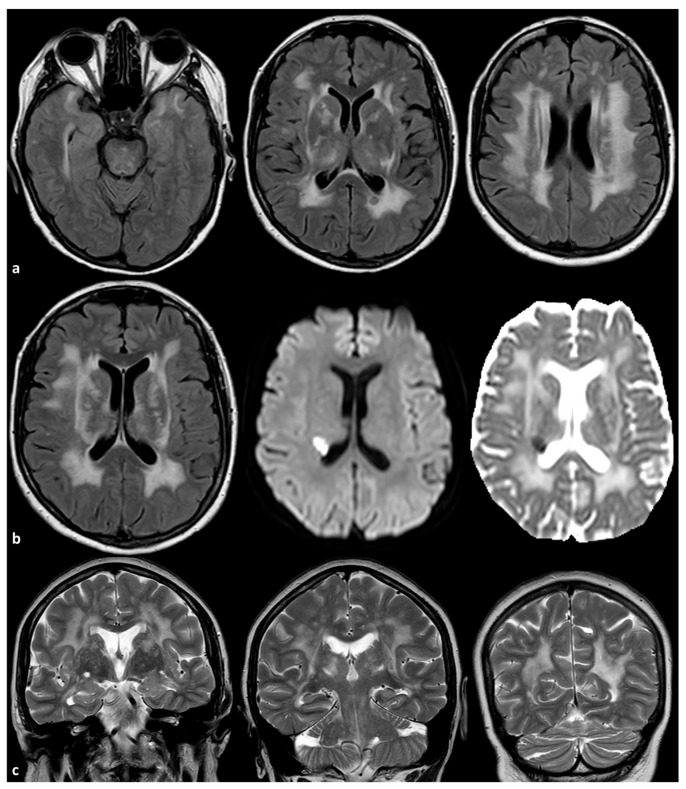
Brain MRI of a 56-year-old woman with a mutation ABCC6 (NM_001351800.1):c.3071G>A:p.(Arg1024Gln) in heterozygosis. The patient had no relevant vascular risk factors and no systemic signs of PXE. In panel (a), axial FLAIR images are provided, showing symmetric, extensive, confluent white matter hyperintensities involving the anterior temporal pole, the external and extrema capsula, the thalamus and the centrum semiovale on both sides (the other known genes responsible for SVD were tested and no mutations were identified). In panel (b), axial FLAIR, axial Diffusion Weighted Imaging and axial Apparent Diffusion Coefficient map images show an acute subcortical ischemia in the corona radiata close to the occipital pole of the right lateral ventricle. In panel (c), coronal T2W images show the enlarged perivascular spaces in the basal ganglia on both sides.
